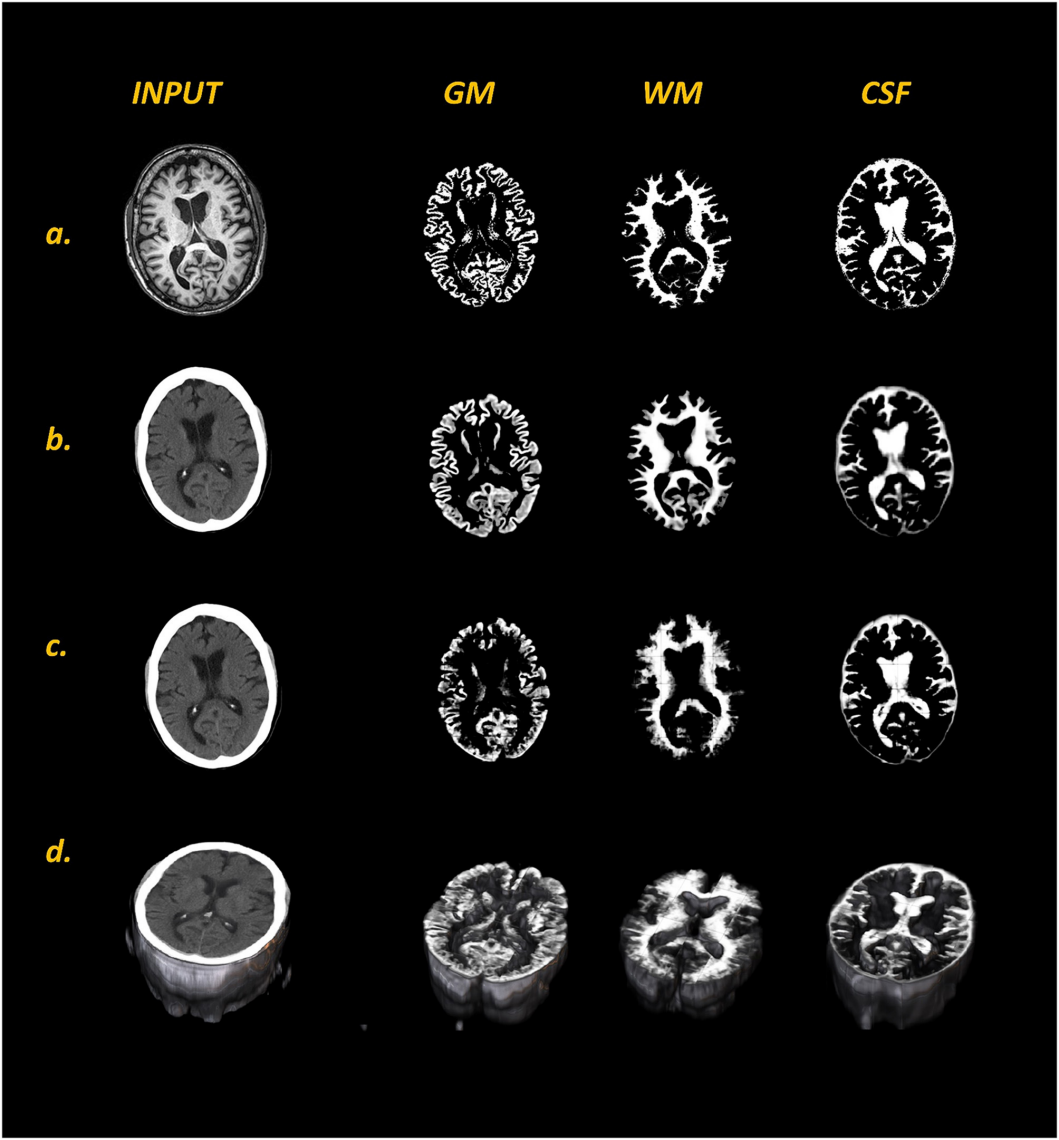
Comparison of MR labels (a) with respective input CT images and predicted tissue class maps (GM, WM, and CSF) generated with 2D U-Net (b) and 3D U-Net (c) models from a representative dataset. Panel (d) shows the 3D visualization of input CT and 3D U-Net predicted tissue class maps. In comparison to 2D U-Nets, 3D U-Nets was not able to resolve the finer details of all three tissue classes, especially WM.
